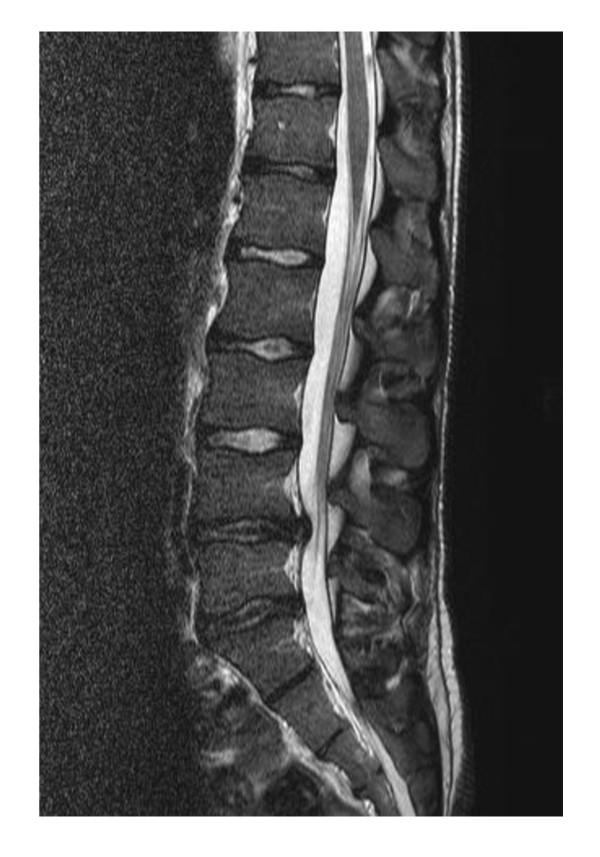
A sagittal T2-weighted MRI image of the lumbar spine showing a centrally located disk herniation at L4/L5 and to a lesser degree at L5/S1. Note the decreased signal of the L4/L5 and L5/S1 disks, indicating decreased disk hydration.
